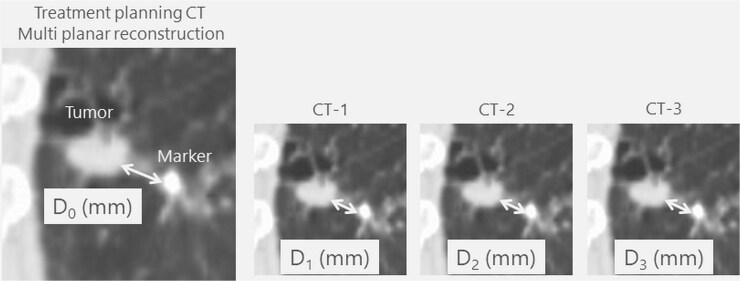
Evaluation methods for measuring relative positions from the marker to the tumor. D_0_, distance from the tumor margin to the marker at treatment planning CT; D1–D3, distances from the tumor margin to the marker in the first to third CT scans, respectively.
